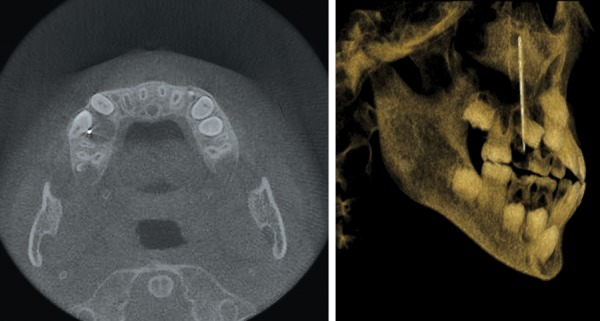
CBCT scan revealed a 4.2 cm long hyperdense image with well-defined margins within the right maxillary sinus, associated with a dentigerous cyst with the crowns of permanent maxillary right second premolar (Tooth 4) germ, the tooth germ displace
